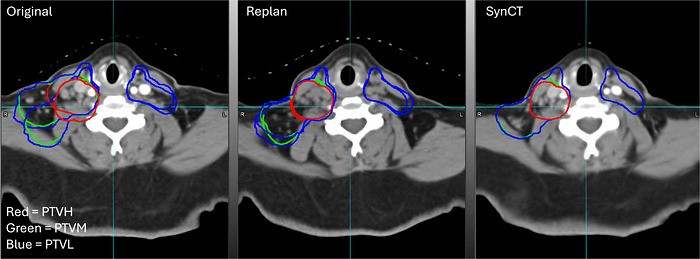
Example of synthetic CT quality assessment comparing the deformed contours to the replan contours for Patient #3. The deformed contours are overlaid with the original contours in left panel, and with the replan contours in middle panel.
